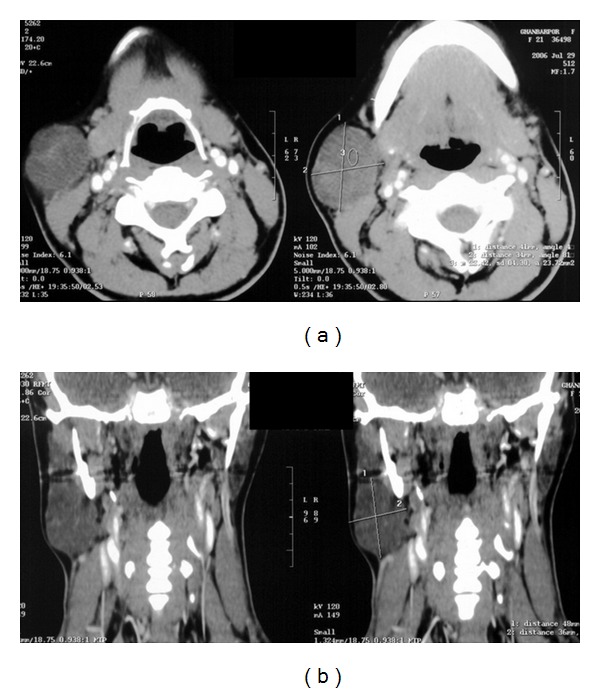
Contrast-enhanced CT scan in axial views of neck. A 36 × 48 mm soft tissue mass shown in the right side of the neck, in association of submandibular salivary gland and extra sheath of carotid, and without any invasion to peripheral tissues.
